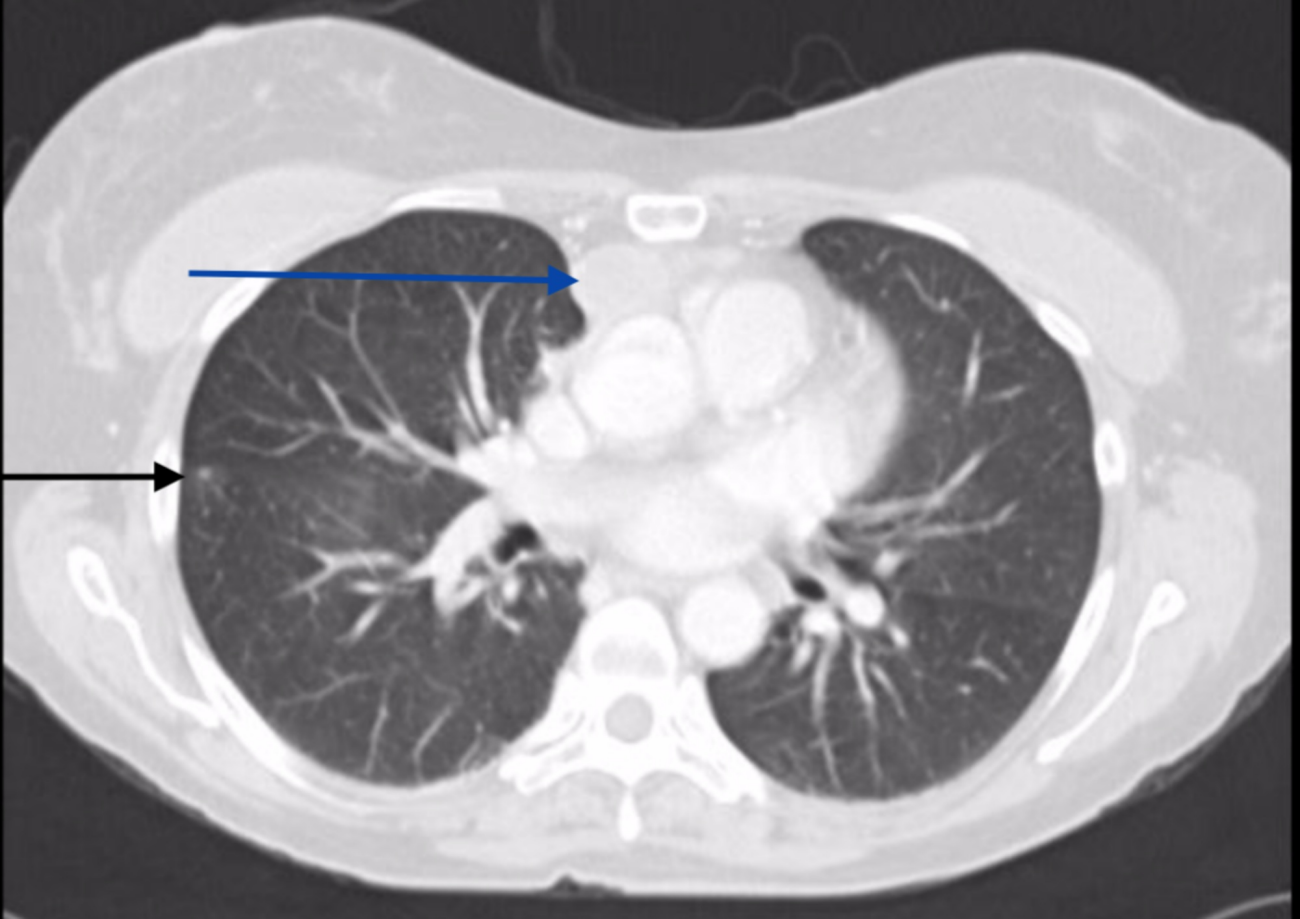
Axial chest CT showing right lung lesion Axial chest CT showing right lung lesion (black arrow) and anterior mediastinal mass (blue arrow).
